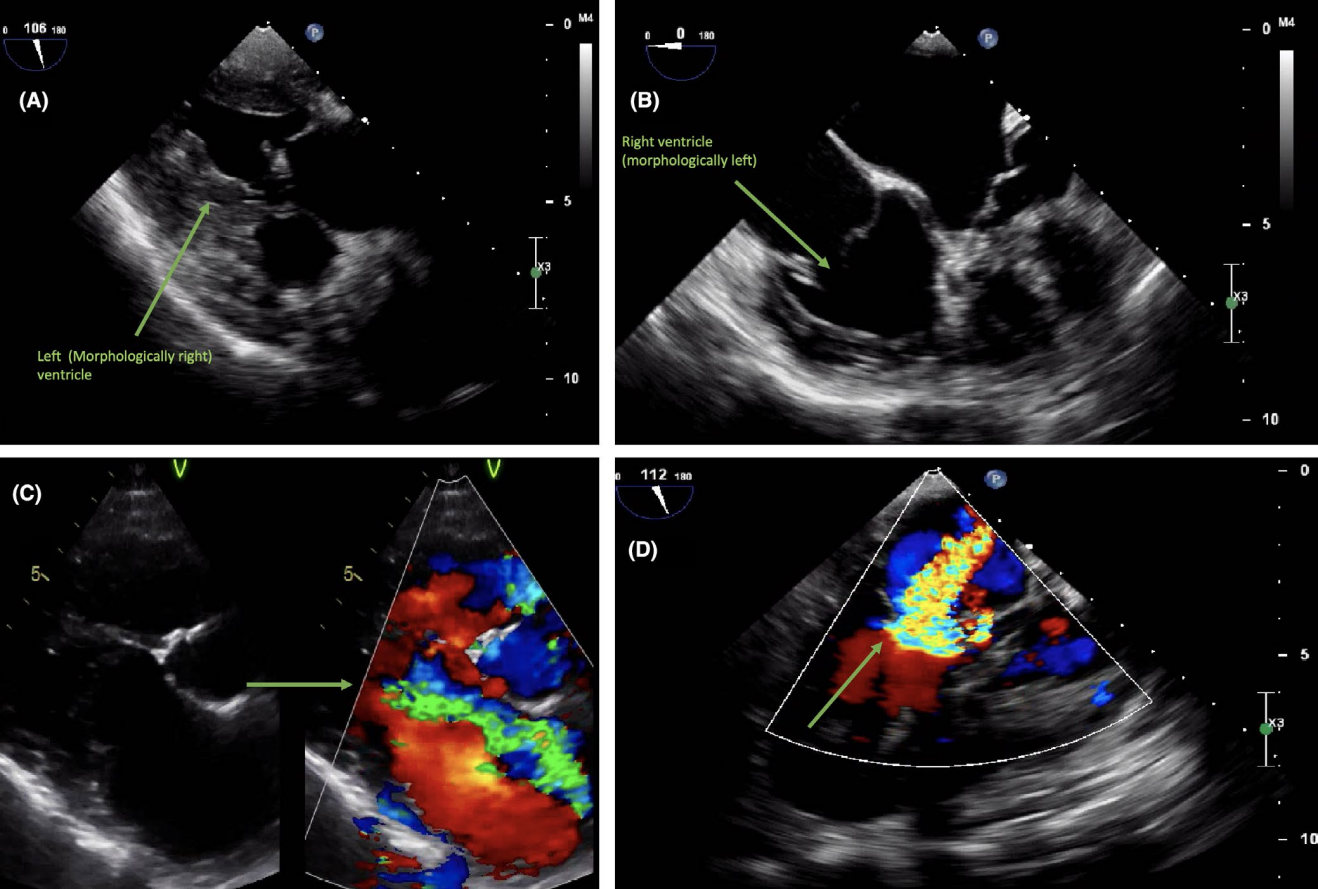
Transesophageal and transthoracic echocardiography views of A, left (morphologically right) ventricular hypertrophy, B, right (morphologically left) ventricular dilatation, and C and D, severe tricuspid (left atrioventricular) valve regurgitation by color‐doppler flow views
